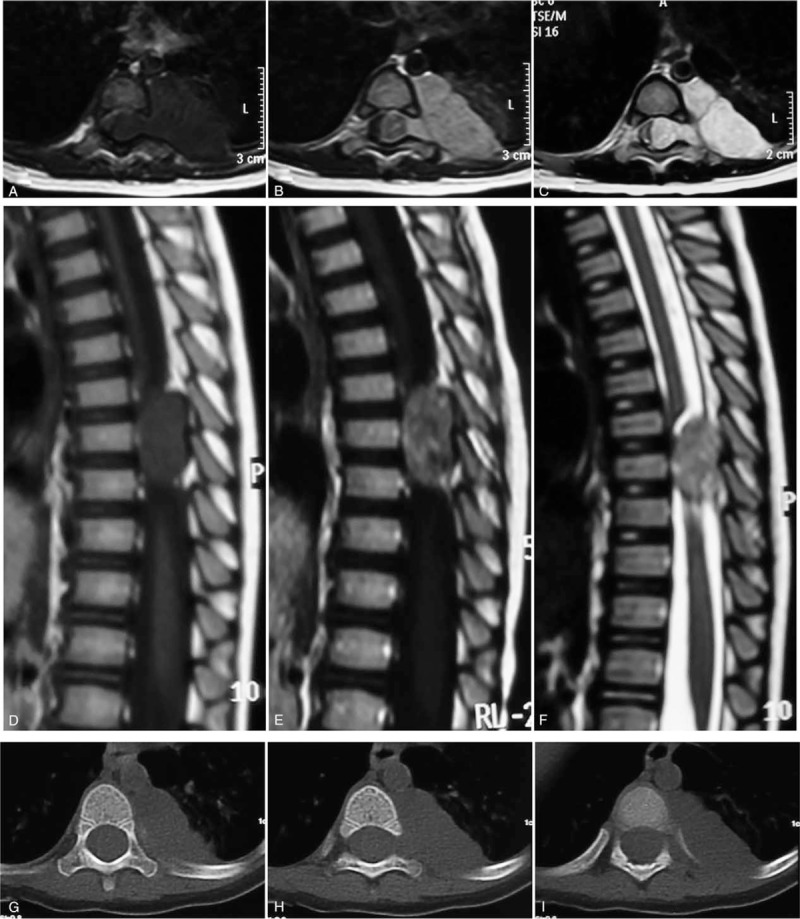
T1-weighted (A, D), Gadolinium-enhanced T1-weighted (B, E), T2-weighted (C, F) MR images, and CT (G, H, I) showing a large extradural and extraspinal mass and marked spinal cord compression at T7–9.
